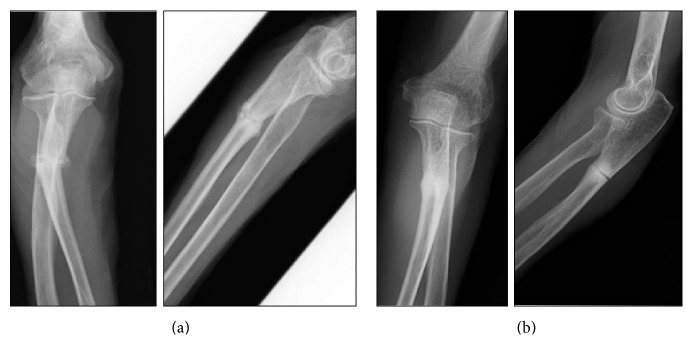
Plain radiographs of the right forearm ((a) case 1 and (b) case 2), showing a transverse fracture with cortical thickening in the proximal third of the ulna suggesting the presence of an atypical ulnar fracture.
